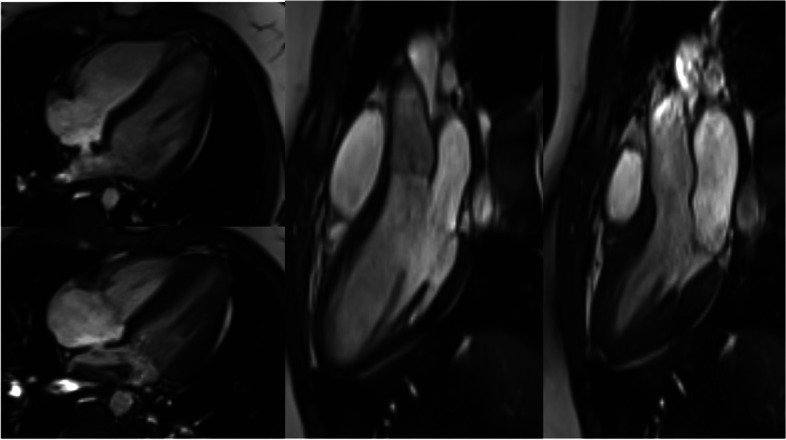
(Functional imaging): assessment of cinematographic (“CINE”) images allows a rapid overview of global and regional functions comparable to echocardiography. Standard are planned projections in the long- and short-heart axis. Here, images of a 12-year-old girl with follow-up after multisystem inflammatory syndrome in children (PIMS) are presented: a 4-chamber view in diastole (left upper row) and systole (left lower row) and a 2-chamber view also in diastole (middle) and systole (right)
